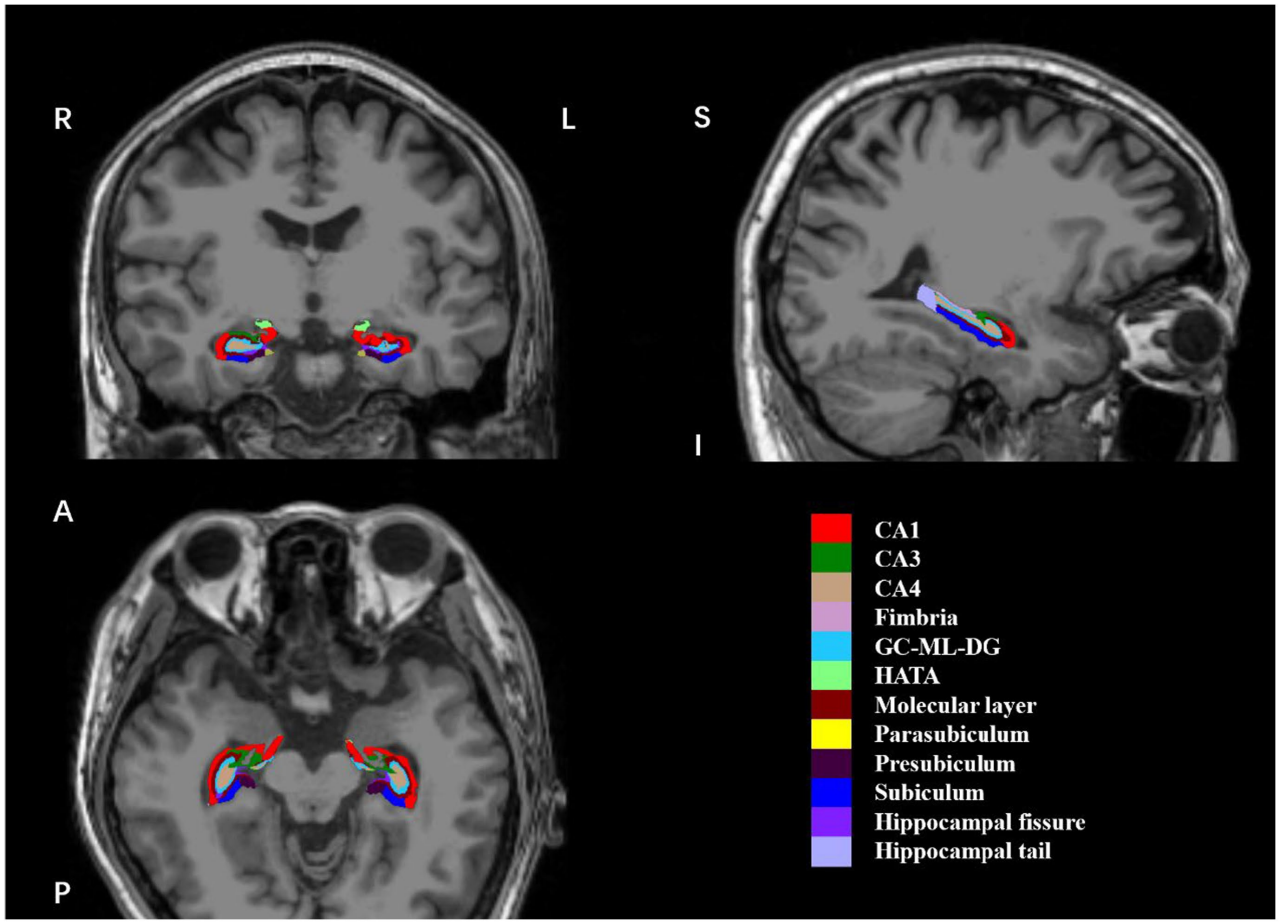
Coronal, sagittal and axial views of 12 hippocampal subfields. The hippocampal subfield volumes were overlaid on whole-brain T1-weighted images in a specific participant in this study. These images were displayed using FreeView. R, right; L, left; (S), superior; I, inferior; (A), anterior; (P), posterior; CA, cornu ammonis; GC-ML-DG, granule cells in the molecular layer of the dentate gyrus; HATA, hippocampal-amygdaloid-transition-area.
